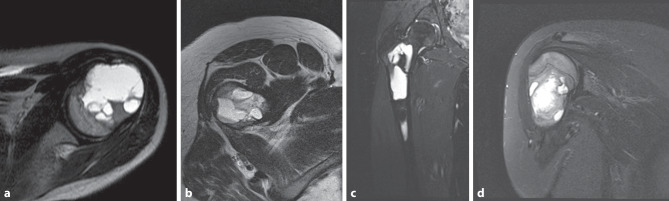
Examples of multichambered UBC in preoperative MRI. a Axial MRI-image of a proximal humeral UBC, b axial MRI-image of a proximal femoral UBC, c frontal MRI-image of a proximal femoral UBC, d frontal MRI-image of a proximal humeral UBC
